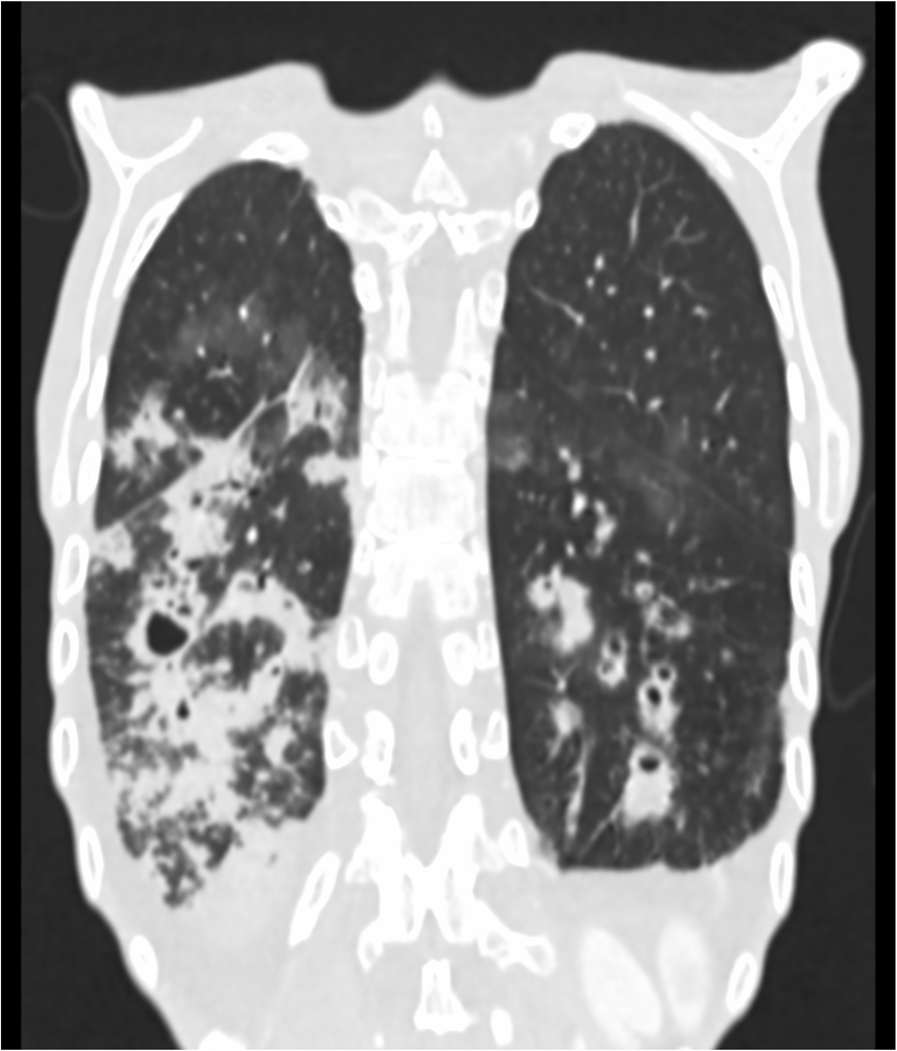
A CT scan of the chest revealed massive, cylindrical and saccular bronchiectases, with some segments of the bronchi filled with fluid content. Furthermore, diffuse areas of ground-glass opacification were also observed
